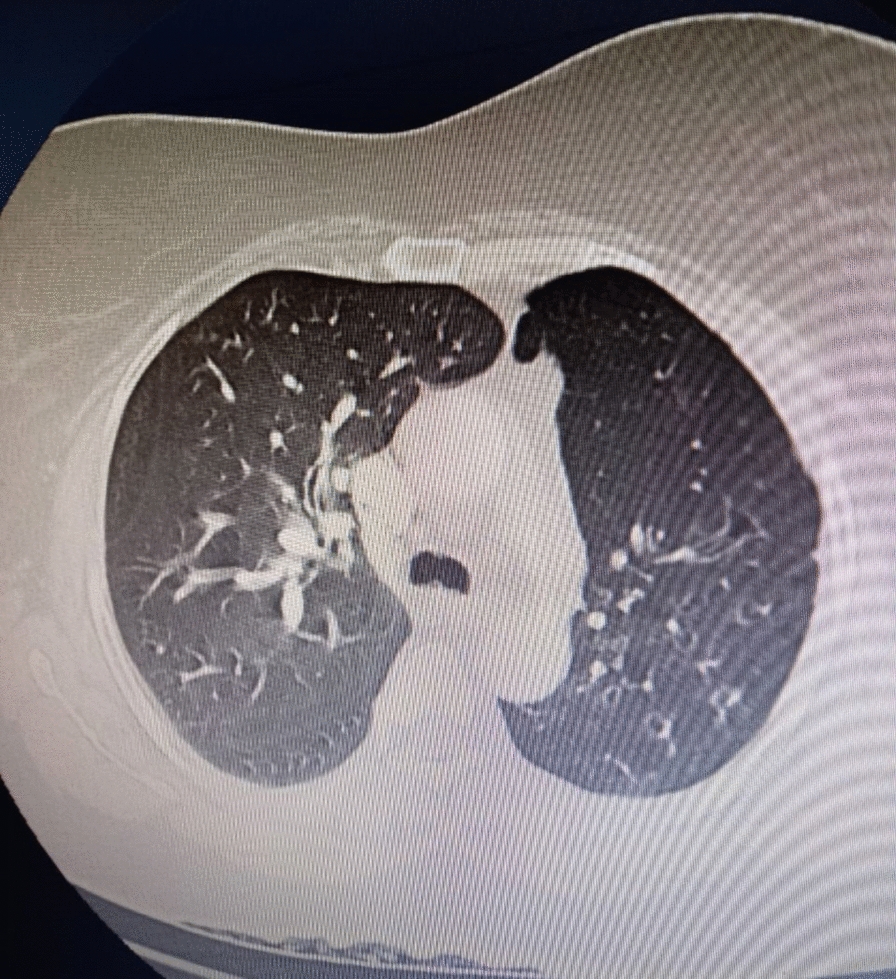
Chest computed tomography showed left lung hyperluceny and bronchiectasis
